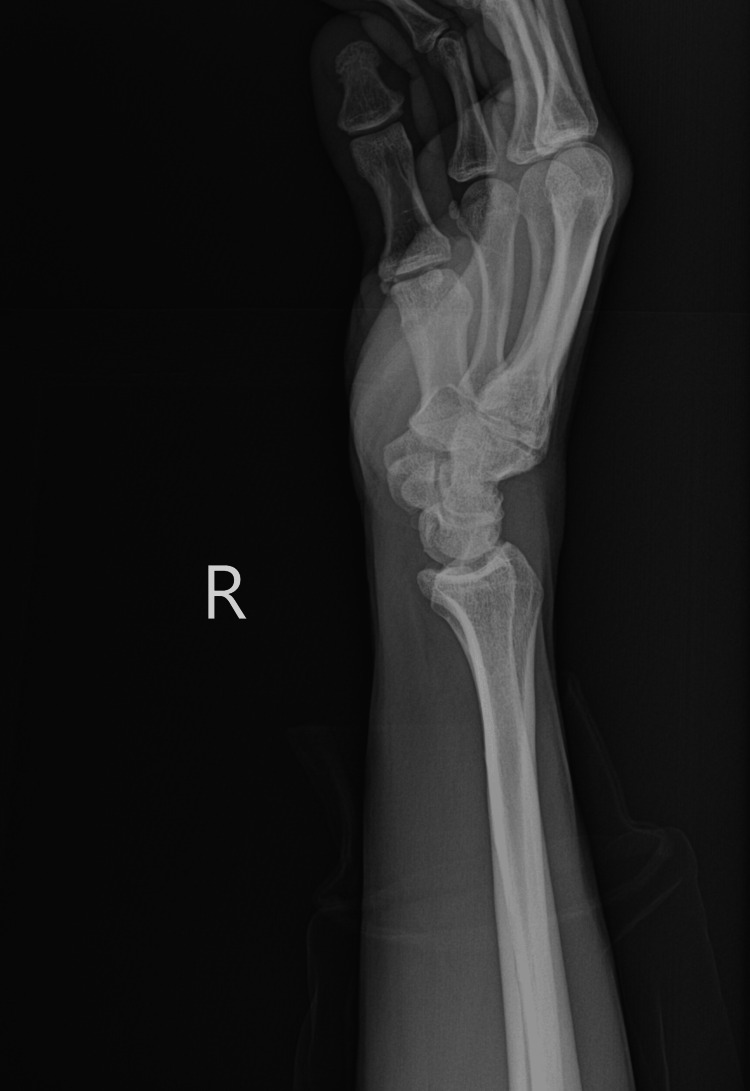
Lateral View Right Wrist X-ray
